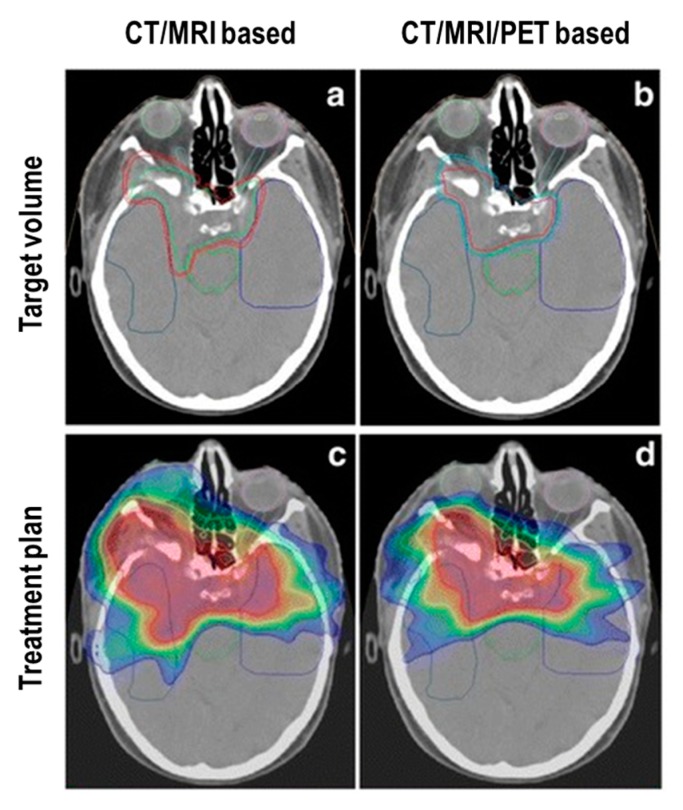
Reduction of IMRT target volume provided by adding 68Ga-DOTATOC-PET to CT and MRI. Panel (a) and (b) display the target volume based on CT and MRI and CT, MRI as well as 68Ga-DOTATOC-PET, respectively in a patient treated for a skull base meningioma. Panel (c) and (d) show the corresponding IMRT treatment plans. The present case shows the reduction of dose to OAR by adding SSTR-PET imaging to treatment planning. *Adapted with permission from Stade et al.* [74].
